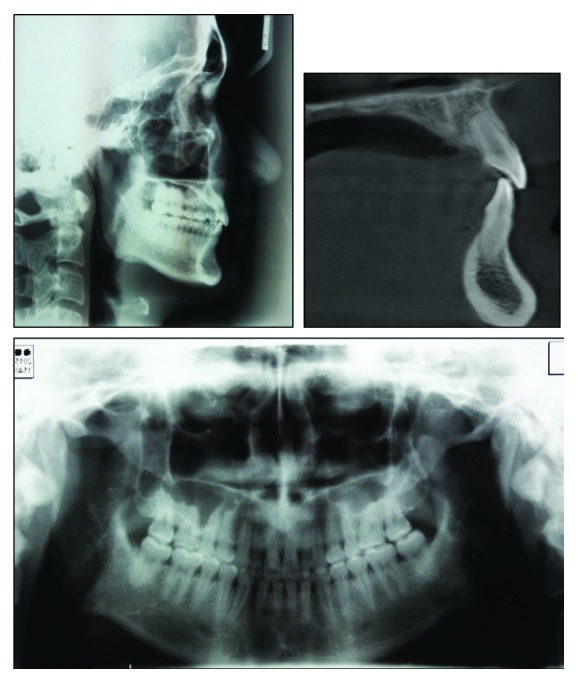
Posttreatment cephalograph, panoramic radiograph, and posttreatment image of maxillary left central incisor from CBCT.
